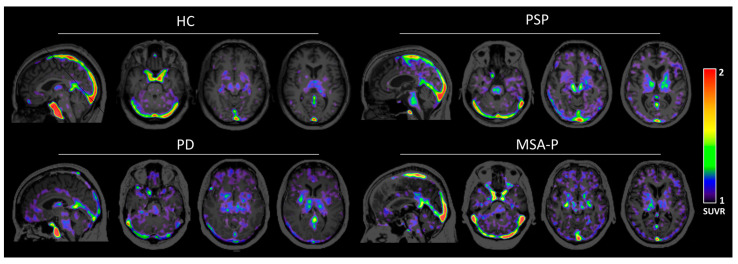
Representative 15–35 min [^11^C]HY-2-15 SUVR image with a whole brain mask of HC, PSP, PD, and MSA-P in the sagittal and axial views of cerebellum, midbrain, and basal ganglia. HC, healthy control; PSP, progressive supranuclear palsy; PD, Parkinson’s disease; MSA-P, multiple system atrophy—parkinsonian subtype; and SUVR, standardized uptake value ratio.
